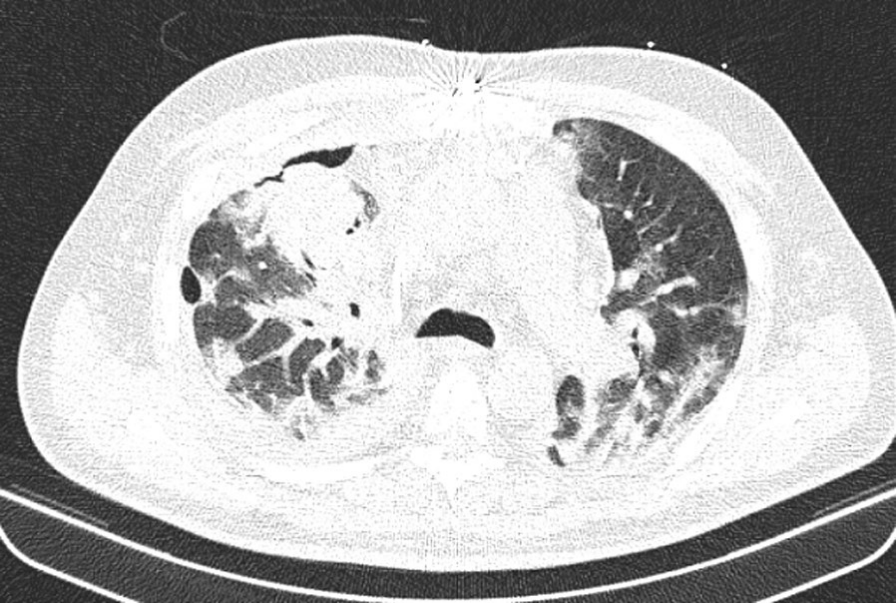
CT image of the lungs, axillary view, showing bilateral opacity
